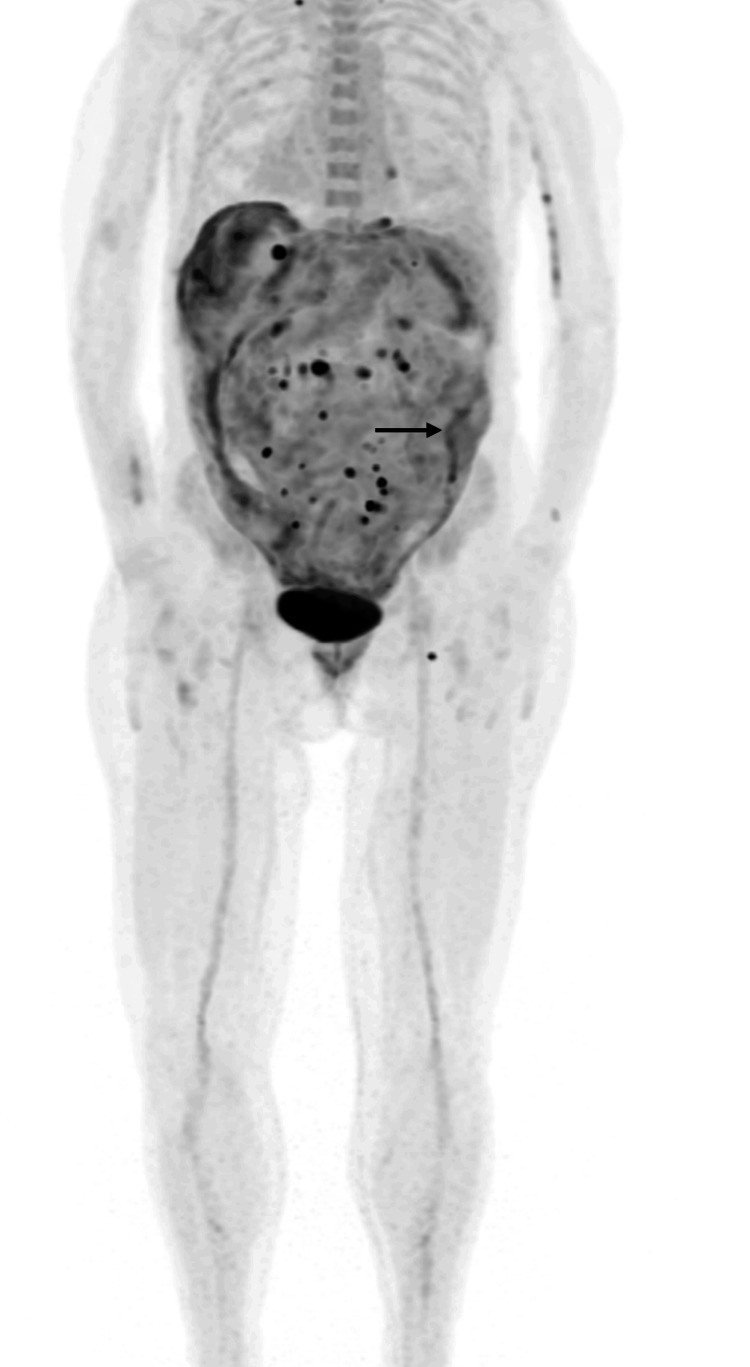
18F-fluoro-deoxy-glucose positron emission tomography (18F-FDG-PET) findings. Several hypermetabolic signs (black dots) in infradiaphragmatic adenopathies and in peritoneal layers (arrow) were found.
